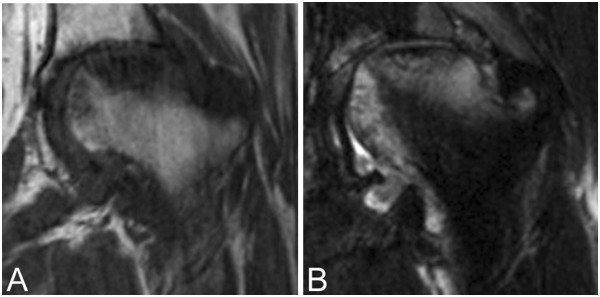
Magnetic resonance images show a bone marrow edema pattern. (A) Coronal T1-weighted magnetic resonance (MR) image shows a diffuse low-intensity signal in the proximal portion of the femoral head. (B) T2-weighted fat-suppression MR image shows diffuse high-intensity signals in both the proximal and medial portions of the femoral head.
